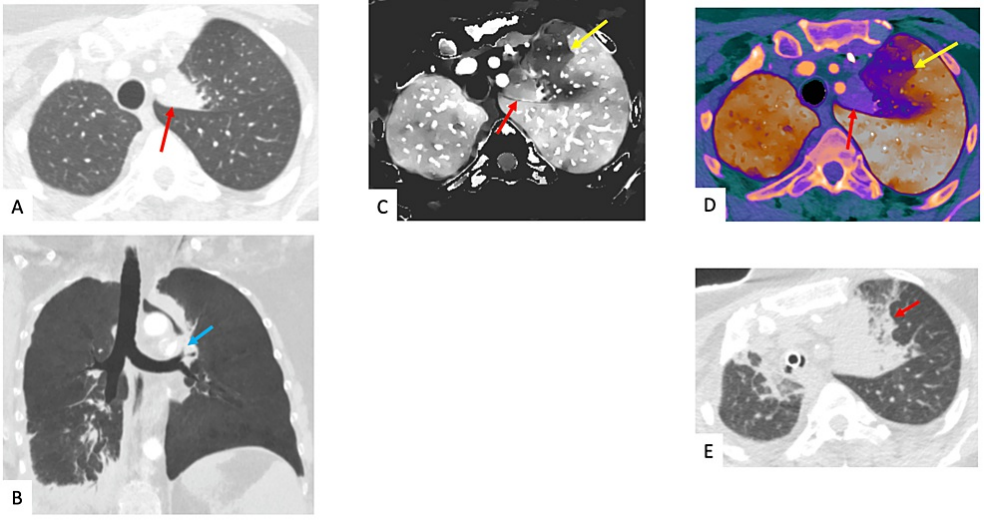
A 54-year-old female with a history of metastatic breast cancer presenting for follow-up chest CT imaging, status post radiation therapy to left mediastinal lymph node. Axial and coronal CT images in lung window setting (A, B) demonstrate a new severe stenosis/obliteration of left upper lobar segmental bronchus (blue arrow) with associated distal postobstructive atelectasis within the medial left upper lobe (red arrow). In corresponding iodine density maps (C) and overlay (D) images, there is demonstration of a geographical perfusion defect within the left upper lobe (yellow arrows) lateral to the homogeneously enhancing atelectasis (red arrows). This is likely due to hypoxic vasoconstriction and suggestive of the area at risk for impending volume loss/atelectasis. Subsequent follow-up chest CT scan (E) within three months confirms the above suspicion and demonstrates increasing left upper lobar atelectasis (red arrow), now also encompassing the area of the previously noted perfusion defect. CT: Computed tomography
